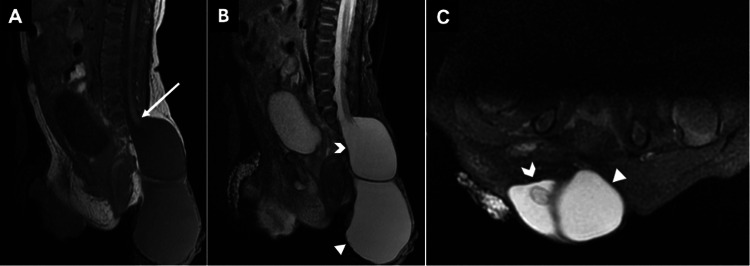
Sagittal T1 (A) and STIR (B) images of the abdomen and pelvis demonstrate a low-lying tethered conus (white arrow) with a surrounding terminal cystocele (white chevron). An additional well-defined fluid-filled structure caudal to the terminal cystocele represents a meningocele (white arrowhead), together with the terminal cystocele comprising a terminal myelocystocele. (C) Axial STIR MR image from the same patient shows the terminal cystocele (white chevron) containing the low-lying tethered conus and the adjacent myelocele (white arrowhead). STIR, short tau inversion recovery All images were obtained by the authors from the institutional picture archiving and communication system (PACS). They have been de-identified and used in compliance with institutional policies.
